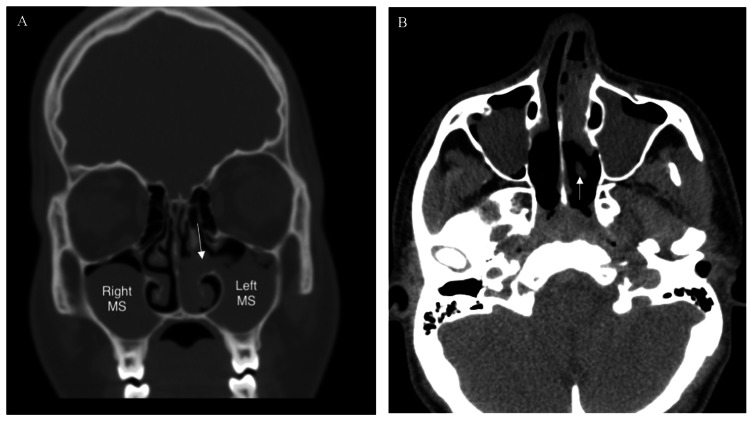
Pre-Operative Imaging. (A) Coronal CT of paranasal sinus showing multiple polypoidal densities occupying the maxillary sinuses bilaterally. (B) Axial CT of the same patient. Arrows: Left antro-choanal polyp S: maxillary sinus.
